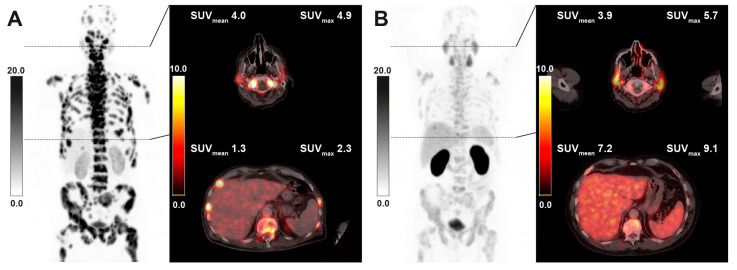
Sample [^68^Ga]Ga-PSMA-11 PET/CT images of a 65-year-old patient with diffuse bone marrow involvement receiving 6 cycles of RLT with cumulative 53.5 GBq of [^177^Lu]Lu-PSMA-617. Maximum intensity projection (MIP) images (left) with axial fusion images showing the parotid gland and liver uptake at baseline (A) and after RLT (B). The decrease in tumor burden is associated with markedly increasing liver uptake, while salivary uptake remains nearly unchanged.
